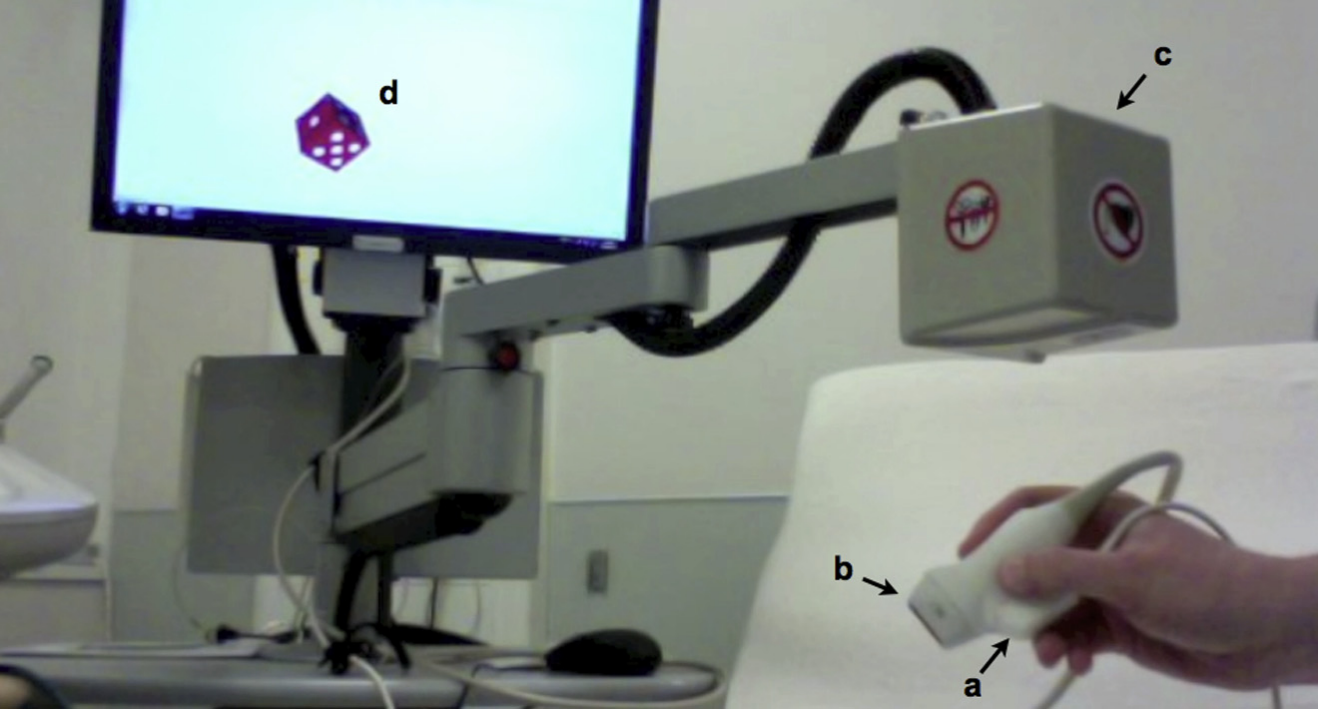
Two-dimensional KBR apparatus: a localizing transducer (A) attached by a molded plastic sheath to a conventional 2D echocardiographic probe (B) detects orthogonal magnetic fields emitted by the generator (C) attached to the mechanical arm that hangs over the patient. Here in the 2D KBR calibration module, the die on the screen (D) represents the 2D echocardiographic probe, which moves synchronously with any movement of the 2D echocardiographic probe. Note the cushioned wedge in the background that is placed on the echocardiography couch to ensure that the metallic apparatus underneath couch does not interfere with detection of the magnetic fields.
